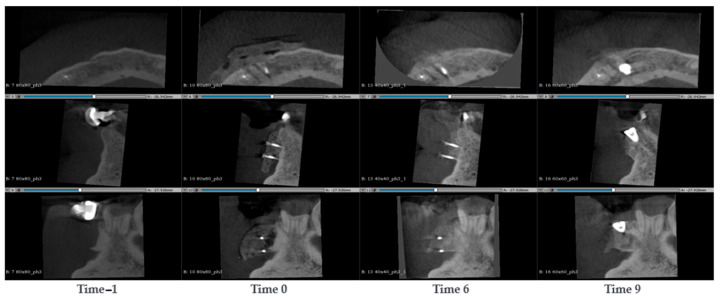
CBCT images of a 59-year-old man with bone resorption around a missing tooth, replaced with a bridge. To recover the bone height, a SmartBone^®^ plate was implanted. The images show the progression of bone healing over time, from pre-surgery (Time −1) to 9 months post-surgery (Time 9).
